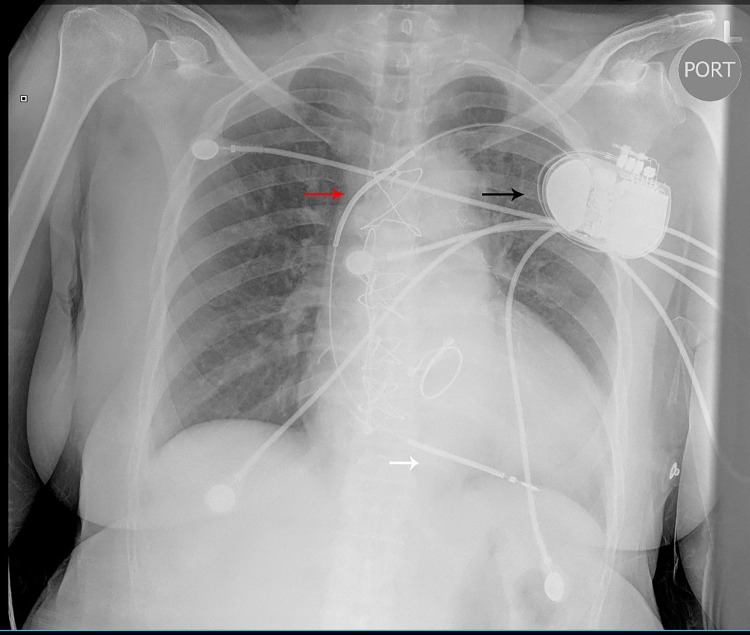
Chest x-ray performed after AICD placement in 2015 Right ventricle lead (white arrow) in the correct place, coil (red arrow) and generator (black arrow). Note its orientation. AICD: automated implantable cardioverter defibrillator
